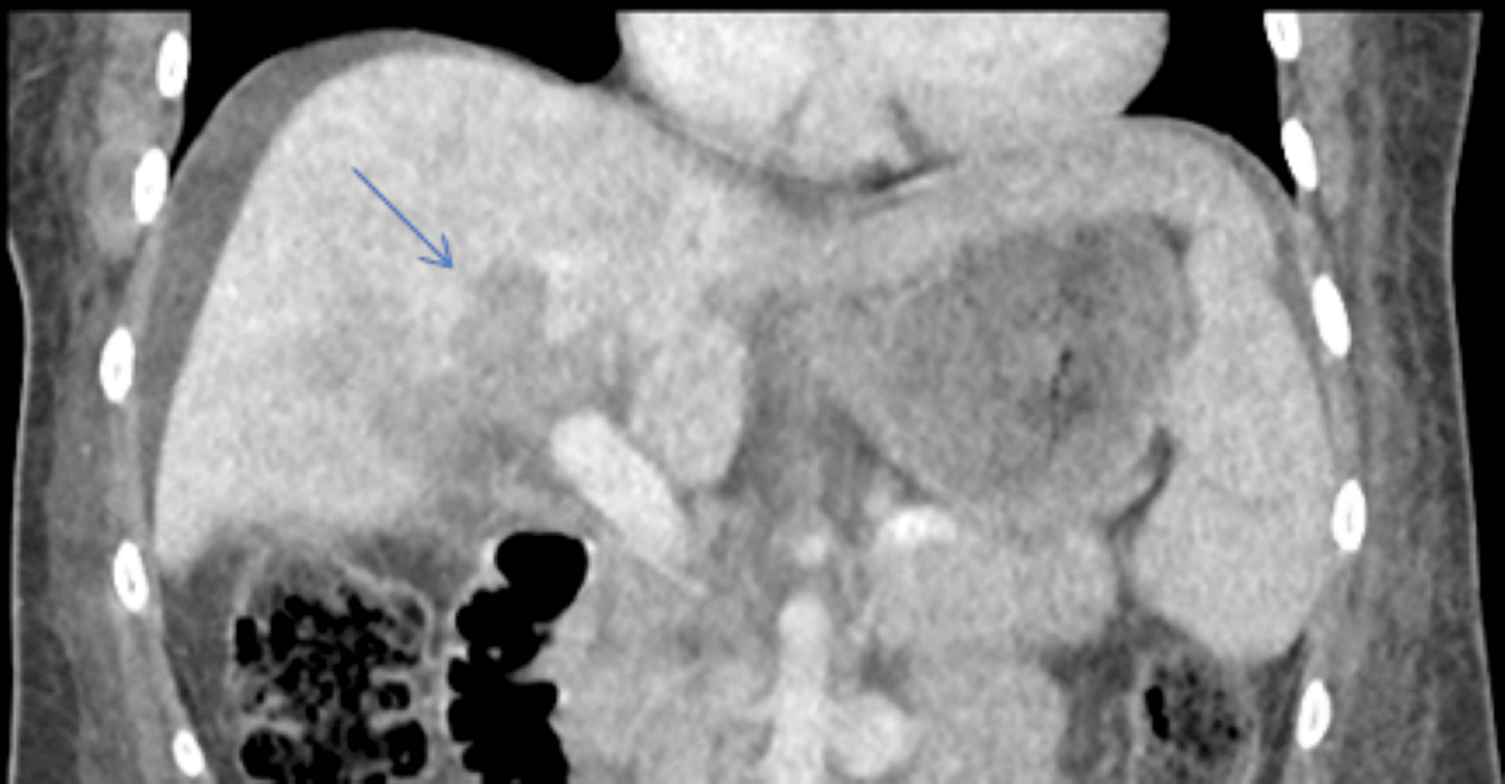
Coronal view of a triphasic CT abdomen pelvis with areas of patchy necrosis indicated by arrow.
